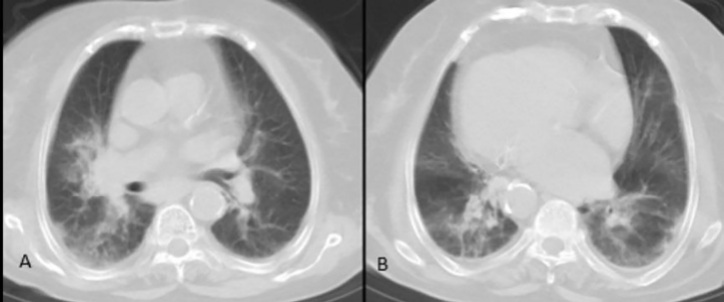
Non-contrast axial chest CT scan of 78-year-old woman Known case of ischemic heart disease showed cardiomegaly, coronary artery calcification, bilateral minimal pleural effusion and para-hilar ground-glass opacities that are typical for cardiopulmonary edema, subsequent echocardiography demonstrated diastolic dysfunction and EF: 35%.
